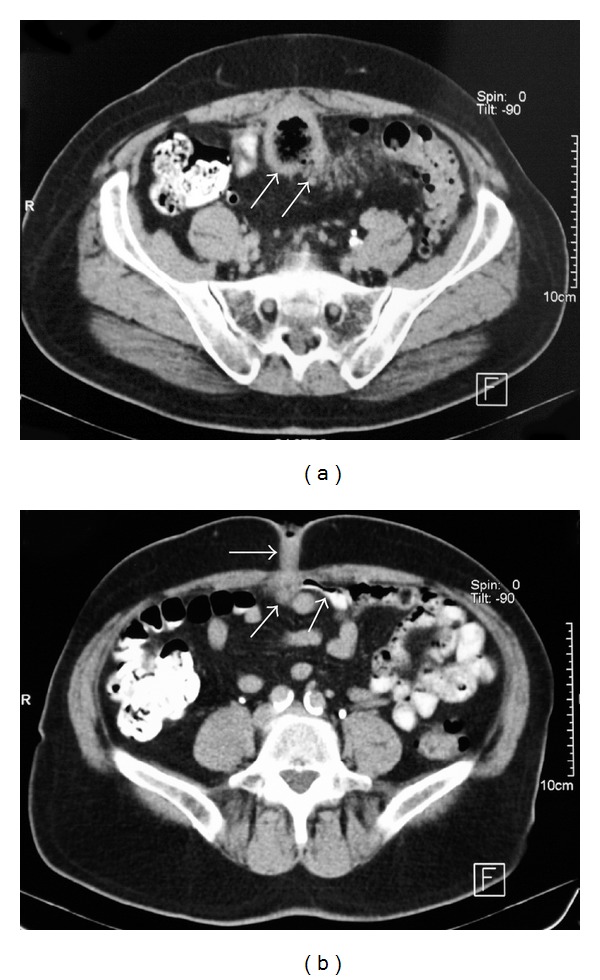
(a) Axial CT scan of the abdomen demonstrating diverticulosis of the sigmoid colon with inflammatory changes in the colonic wall (arrows) and mesenteric stranding with the sigmoid adherent to the posterior aspect of the anterior abdominal wall. (b) A thick-walled cavity (arrows) is present beneath the umbilicus communicating with the sigmoid colon.
